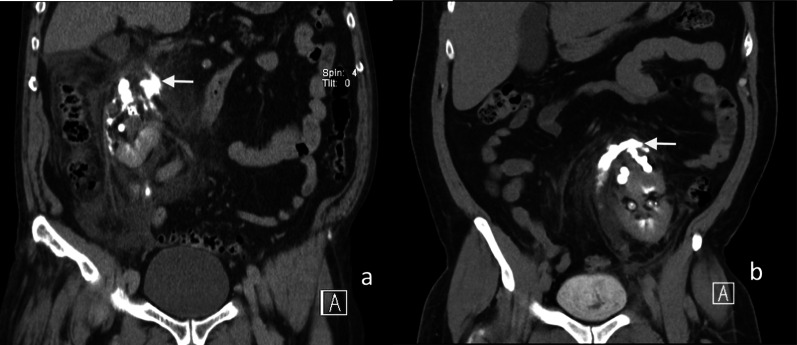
Coronal delayed excretory phase CT images of calyceal rupture and urinoma formation (white arrows) with perinephric extravasation of contrast in two patients. a Post-ESWL and (b) post-pyeloplasty
